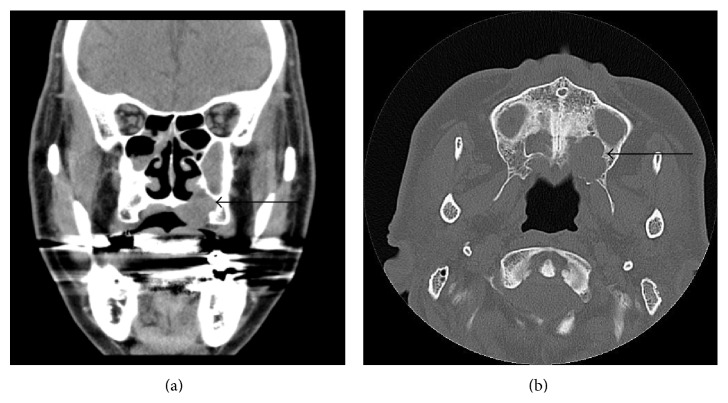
CT images. (a) Frontal plane CE-CT image showing the presence of a 1.8 × 1.4 cm mass in the left hard palate, which extended upward and invaded the nasal cavity (arrow). (b) Horizontal image showing hyperplasia localized in bone (arrow) observed upward and backward to the maxillary sinus. No bone destruction was observed.
